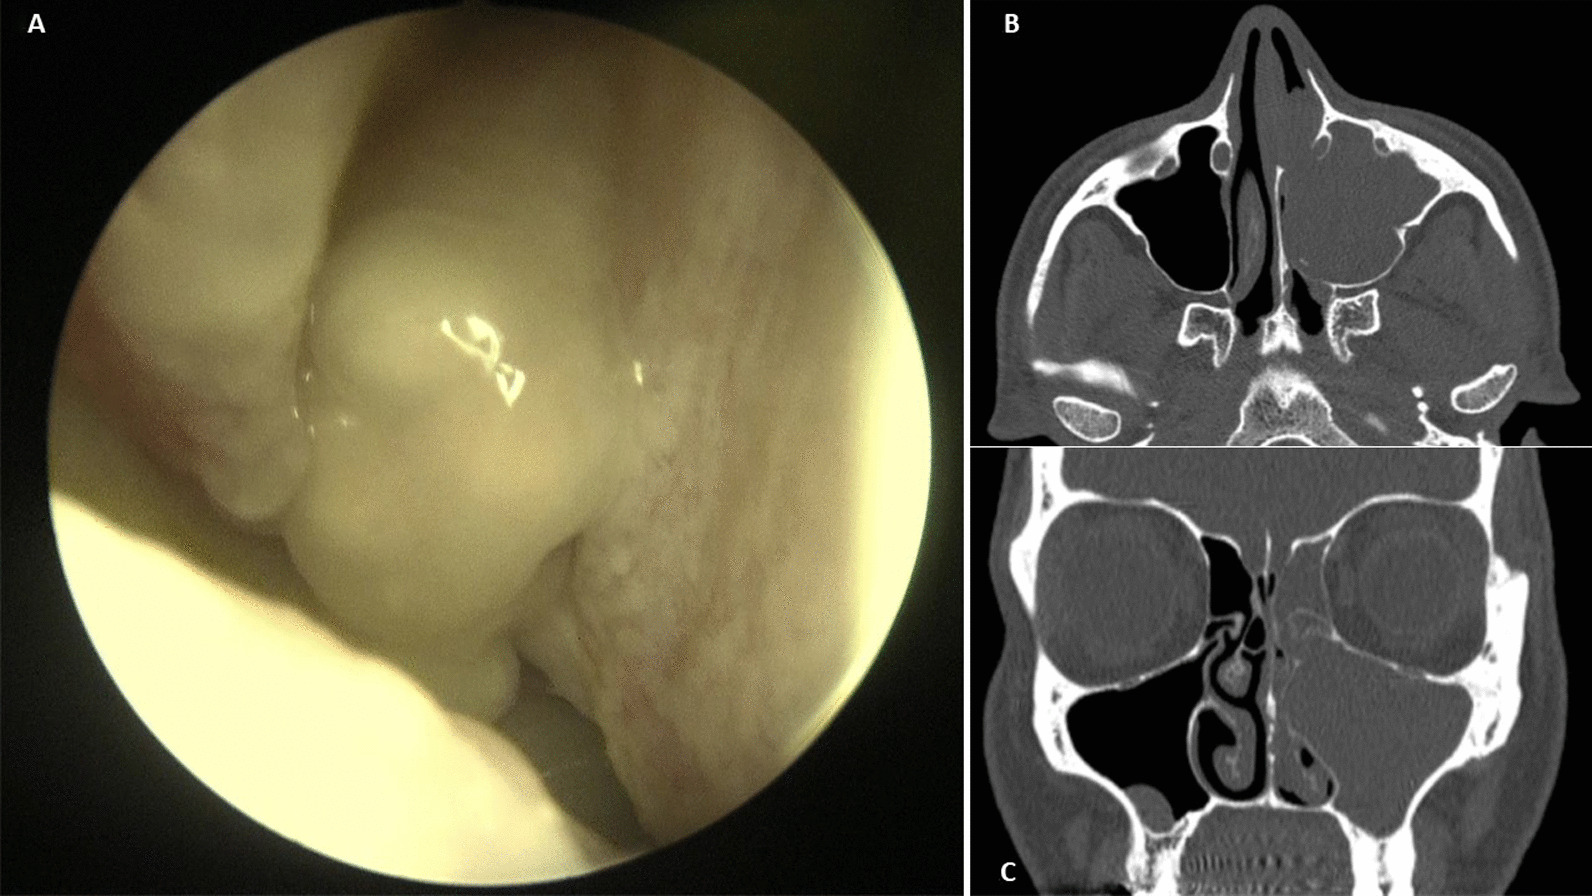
a Endoscopic appearance of a grayish polypoid mass lesion with a multinodular surface occupying the entire left nasal fossa, b Head CT scan: a axial view, c coronal view. Evidence of a lesion, 24 mm × 28 mm × 26 mm in size, totally obliterating the left maxillary sinus, resulting in obstruction of the anterior osteomeatal unit and ethmoidal sinusitis
